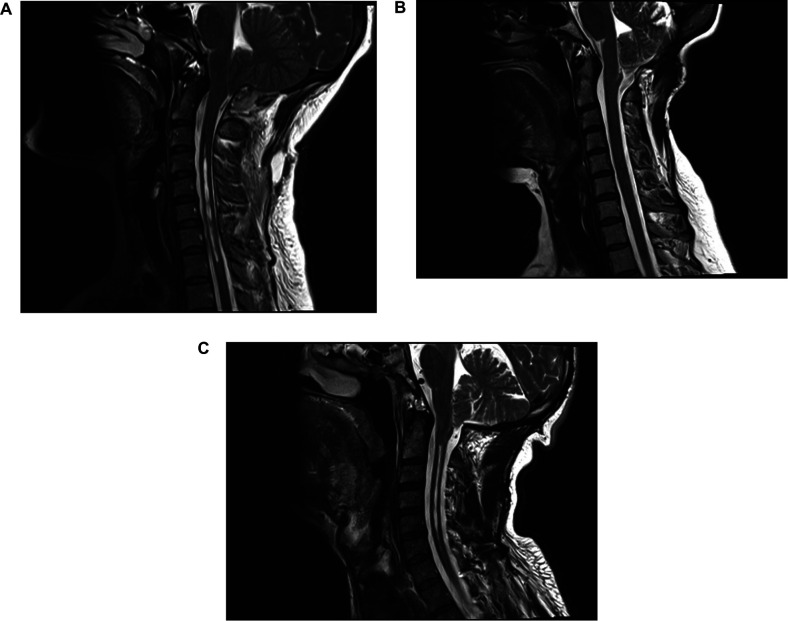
(A) Preoperative sagittal T-2 weighted MRI image of a patient with CM1 with syringomyelia, (B) MRI image taken at the 6th month follow-up after suboccipital craniectomy shows that the syringomyelia is not shrinkage and the crowding at the craniovertebral junction continues, (C) after CTR, the syringomyelia is shrinkage and the CVJ is decompressed.
